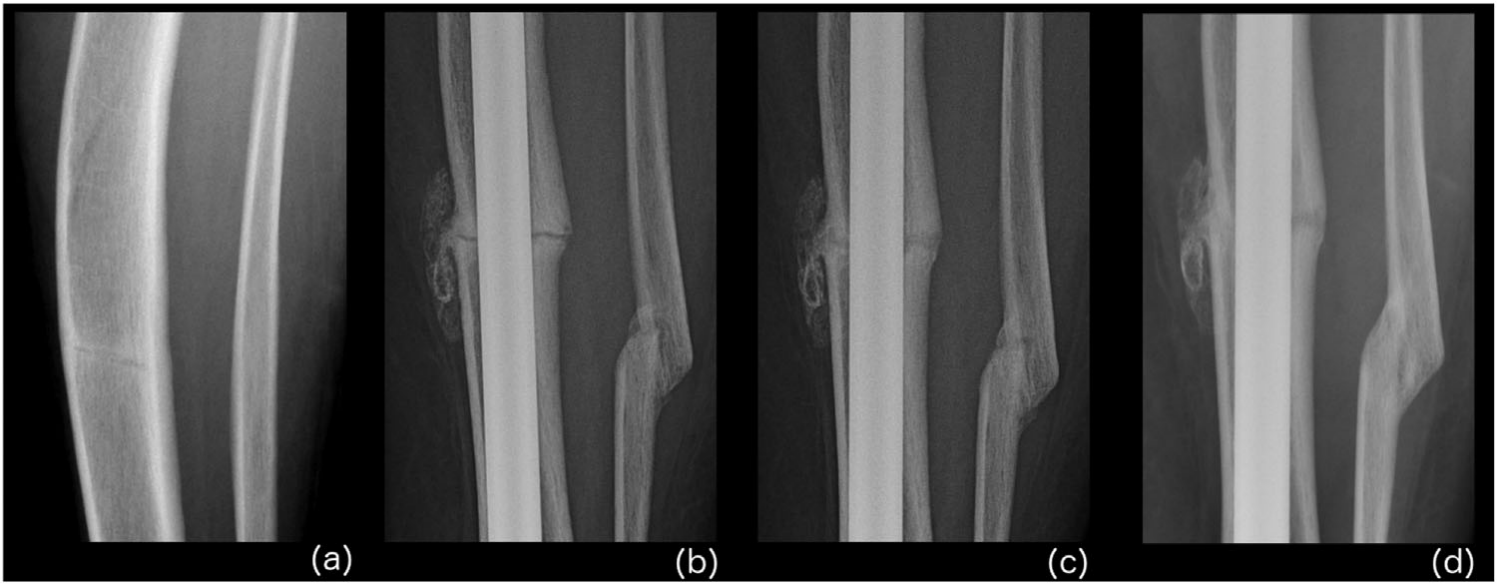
Case 1: Radiograph series of left tibia‐fibula after osteotomy and intramedullary nail (IMN) fixation. (*A*) Three years after left tibial fracture; before replacement therapy and operative treatment. (*B*) One month after elective osteotomy; 2 months after asfotase alfa initiation. Some callus formation at the osteotomy site. (*C*) Five months post‐op; 6 months on medication. Definite increase in callus formation. (*D*) Ten months post‐op; 11 months on medication. Tibial and fibular osteotomies appear to have gone on to union.
